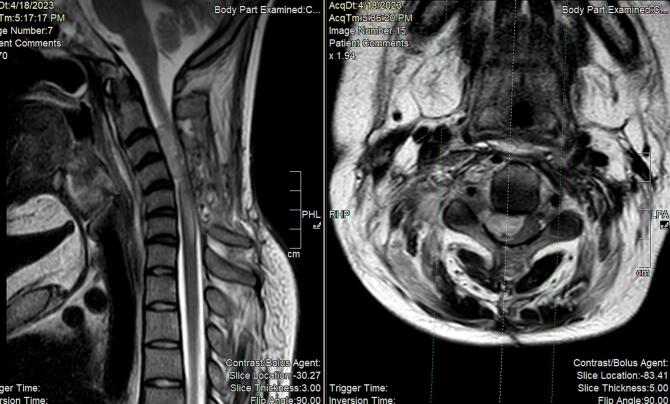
Fig. (4-a) T2 weighted cervical spine MRI sagittal cut showing. Fig. (4-b) T2 weighted cervical spine MRI axial cut showing mass extension along C3-C4 right exit foramen and a new epidural mass measuring 2.9 × 1.5 cm in length along C3 and C4 levels. The mass is causing compression upon the spinal cord at this level.
